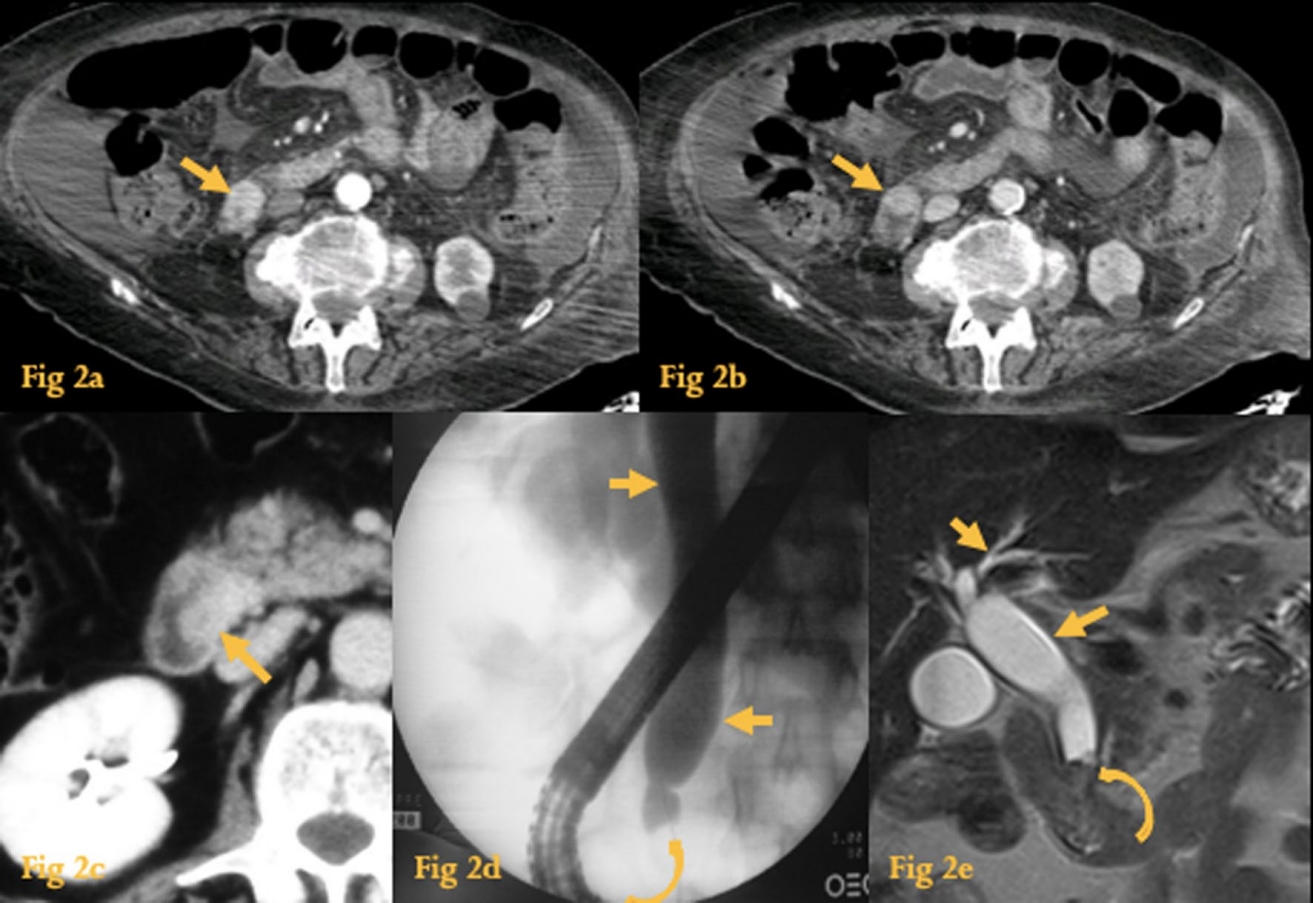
Ampullary tumors. Contrast-enhanced CT (a, b) reveals an enhancing soft-tissue mass (arrows), originating in the medial wall of the descending duodenum. This was a case of an ampullary adenocarcinoma. Contrast-enhanced CT of another patient with an ampullary carcinoma (c), shows a soft-tissue mass (arrow) originating in the medial wall of the descending duodenum and protruding into the water-filled duodenum. ERCP (d) revealed an enlarged and irregular suspicious papilla (curved arrow) and CBD dilatation (arrows). MRCP (e) of another patient shows dilatation of the CBD and intra-hepatic ducts (arrows) due to an abrupt stop at the distal CBD (curved arrow), which was caused by an ampullary villous adenoma
